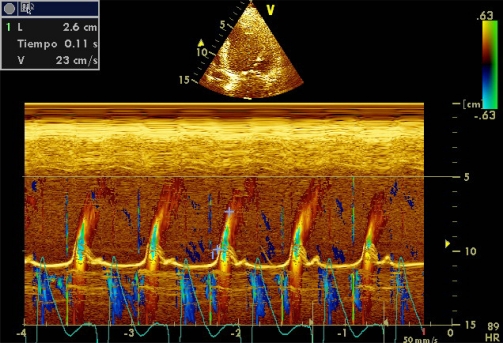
Color M-mode Doppler flow propagation velocity.
